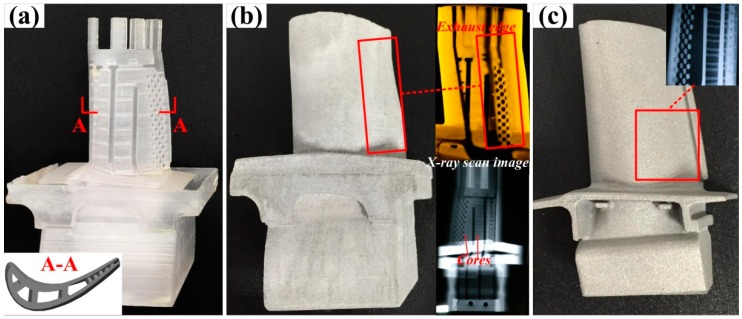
Fabrication of a hollow turbine blade: (a) Resin mold; (b) Mullite whisker-reinforced ceramic mold with X-ray inspection; (c) Hollow turbine blade.
